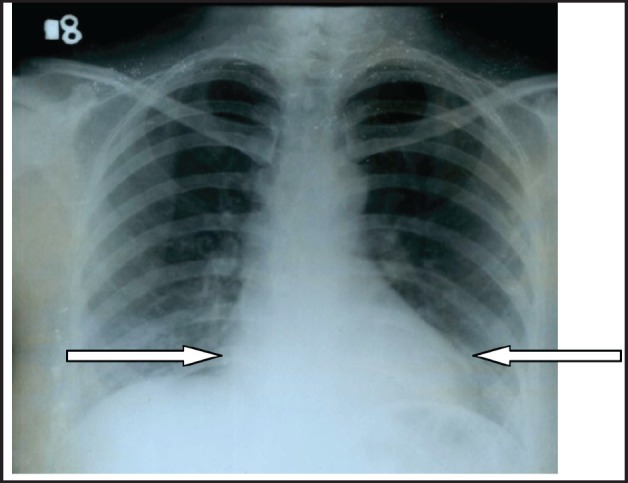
Chest x-ray PA digital view did not reveal any major abnormalities except for marginal increase of cardiac size in transverse diameter.
